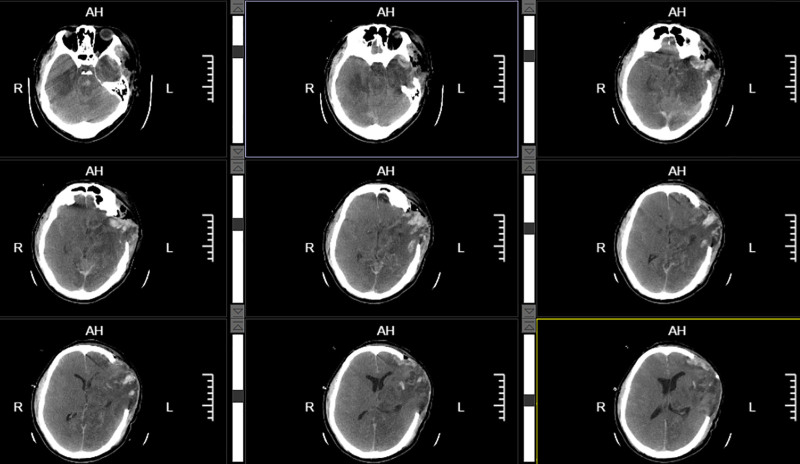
CT imaging revealed obliteration of the ambient cisterns, diffused cerebral edema, compression of the brainstem, and marked signs of intracranial hypertension. CT = computed tomography.
